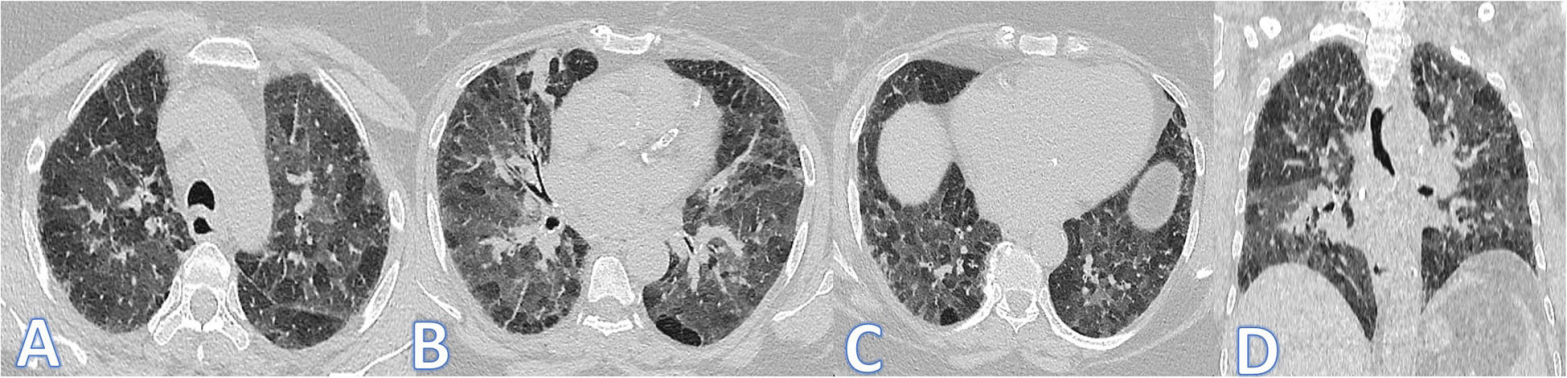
A 64-year-old female axial and coronal CT image shows the headcheese sign, featuring sharp geographic margination along the edges of the secondary pulmonary lobules with three distinct levels of attenuation representing normal, ground glass, and hyperinflated regions of lung. The diagnosis was subacute HP
